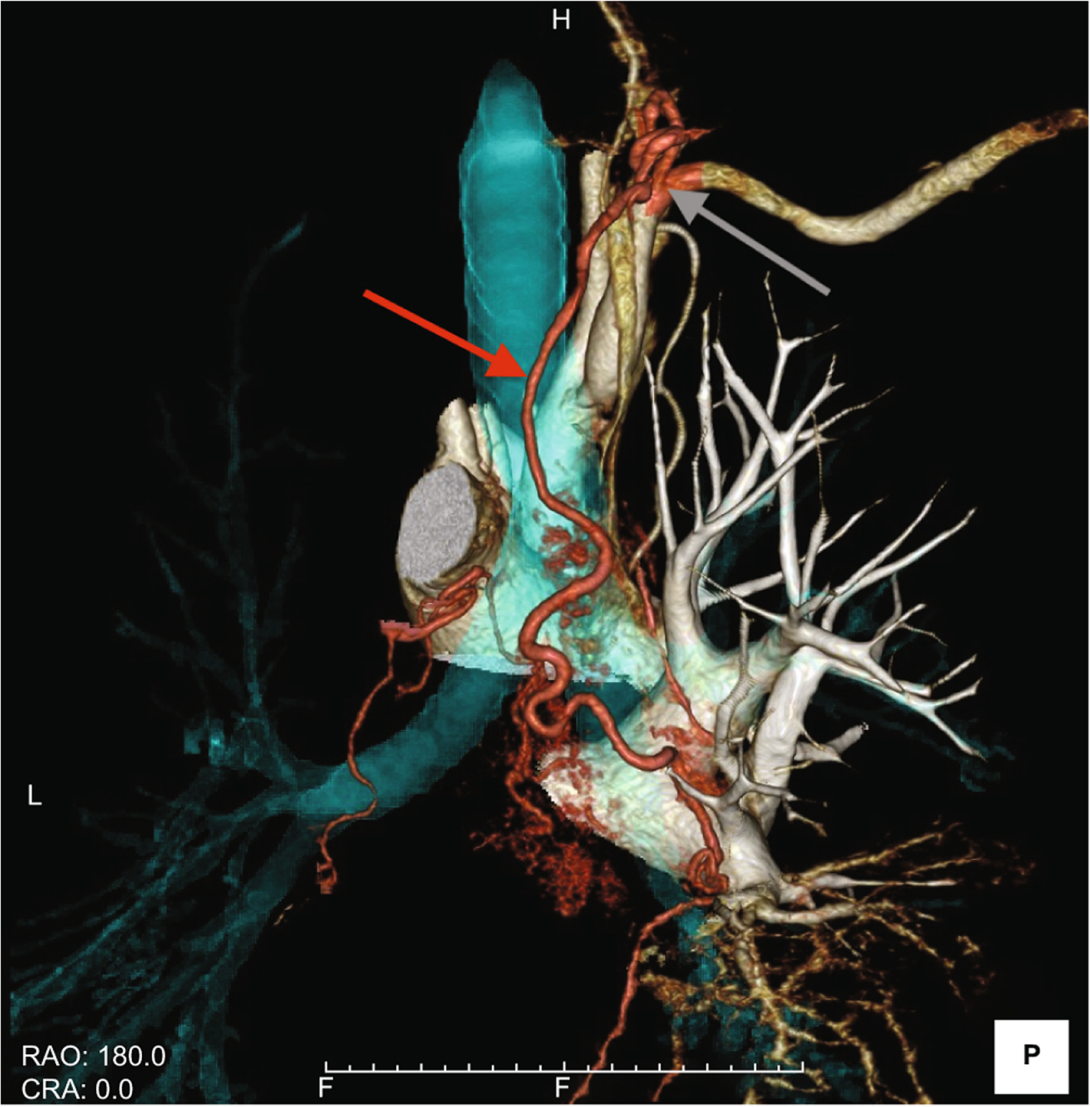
Volume rendering reconstructed image of multidetector computed tomography angiography shows a hypertrophic ectopic right bronchial artery (red arrow) arising from the right thyrocervical trunk (gray arrow)
